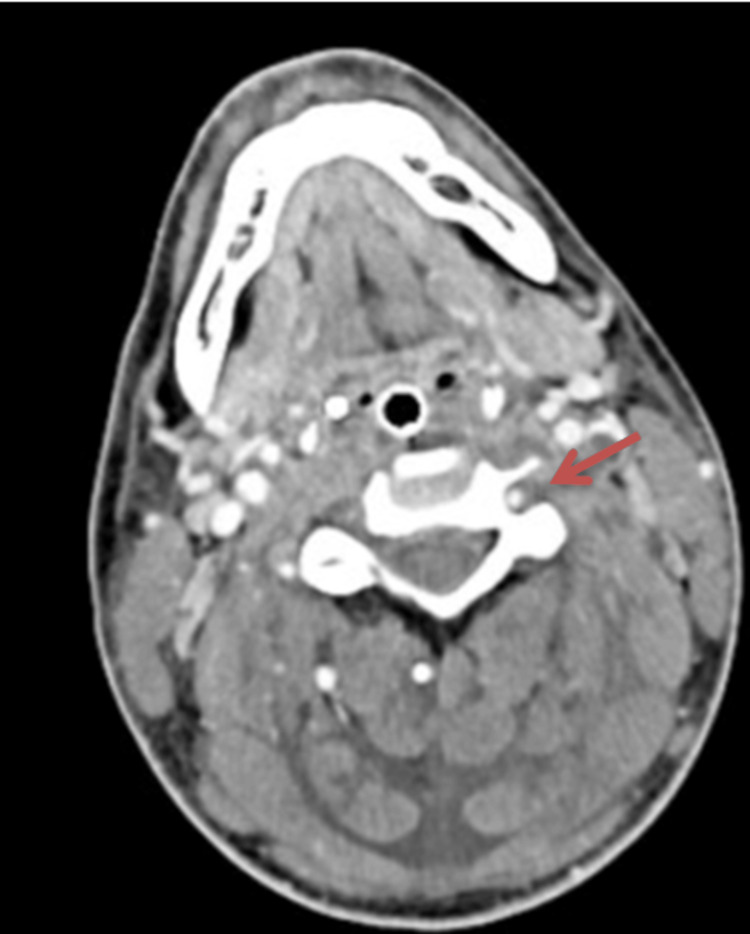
Computed tomography (CT) of the neck. CT of the neck showing a non-occlusive 30-mm-long thrombus in the left internal jugular vein (red arrow).
